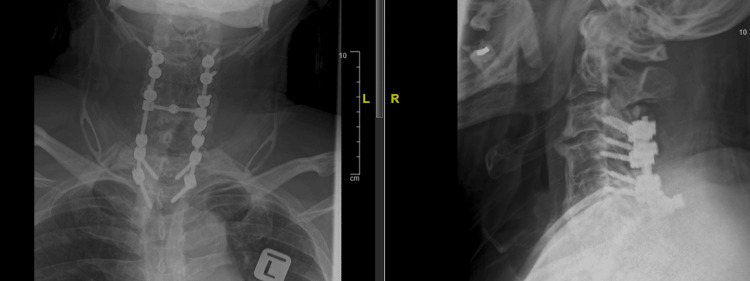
Anteroposterior and lateral cervical radiograph post instrumentation shows instrumentation three levels above and below the C6 vertebrae
